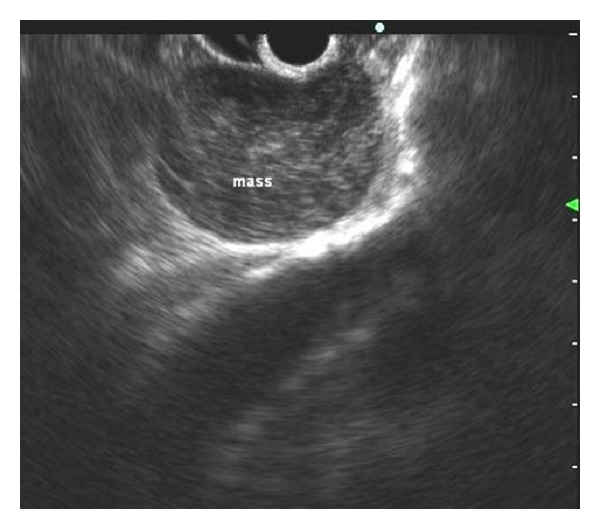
Endoscopic ultrasonography showing a hypoechoic mass with a slightly heterogeneous internal echo and regular margins located just below the gastroesophageal junction.
